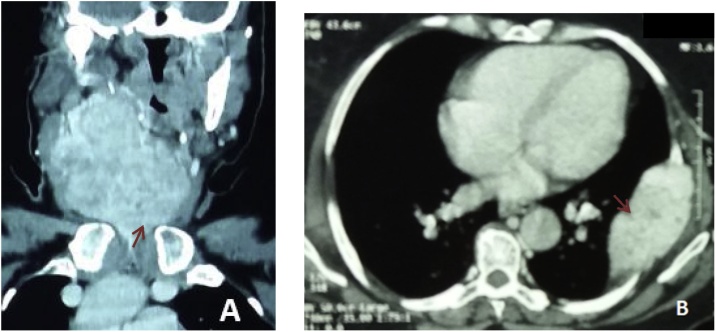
CT images showing the voluminous plunging goiter (A) and its costal metastasis (B).
